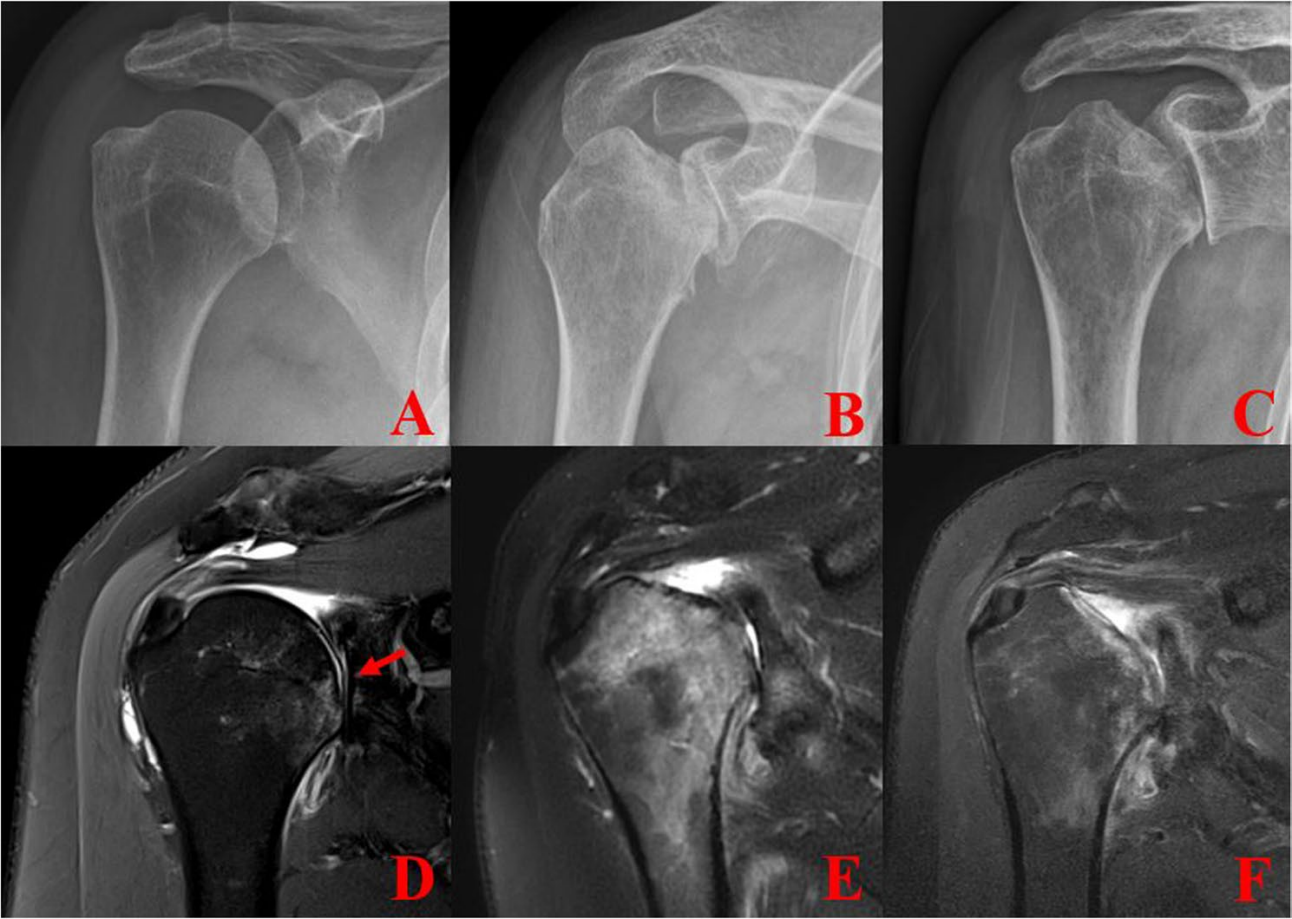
Mid-chondrolysis stage of rapidly progressive destructive arthropathy (RPDA): A, D Initial anteroposterior radiographs of the right shoulder taken at the first visit showed no apparent abnormalities of the humeral head. However, MRI revealed focal bone marrow edema in the humeral head (red arrow). B, E At the second visit, four months later, radiographs demonstrated progressive destruction of the humeral head, and MRI showed partial collapse with extensive bone marrow edema involving the entire humeral head. C, F Three months later, further progressive destruction was noted; however, the collapse did not exceed 50% of the humeral head. Follow-up MRI showed gradual resolution of the bone marrow edema over time
